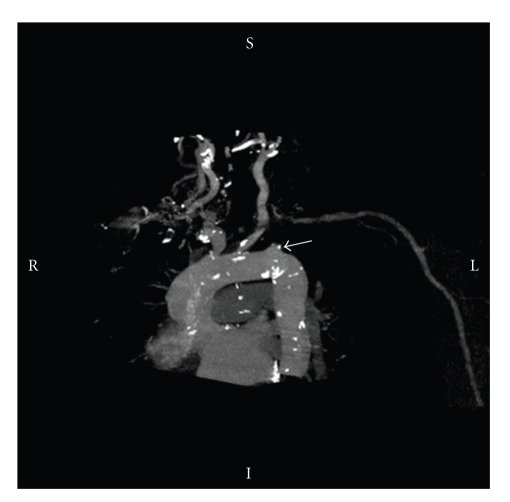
CT angiogram demonstrating the proximal stenosis of the left subclavian artery (arrow).
